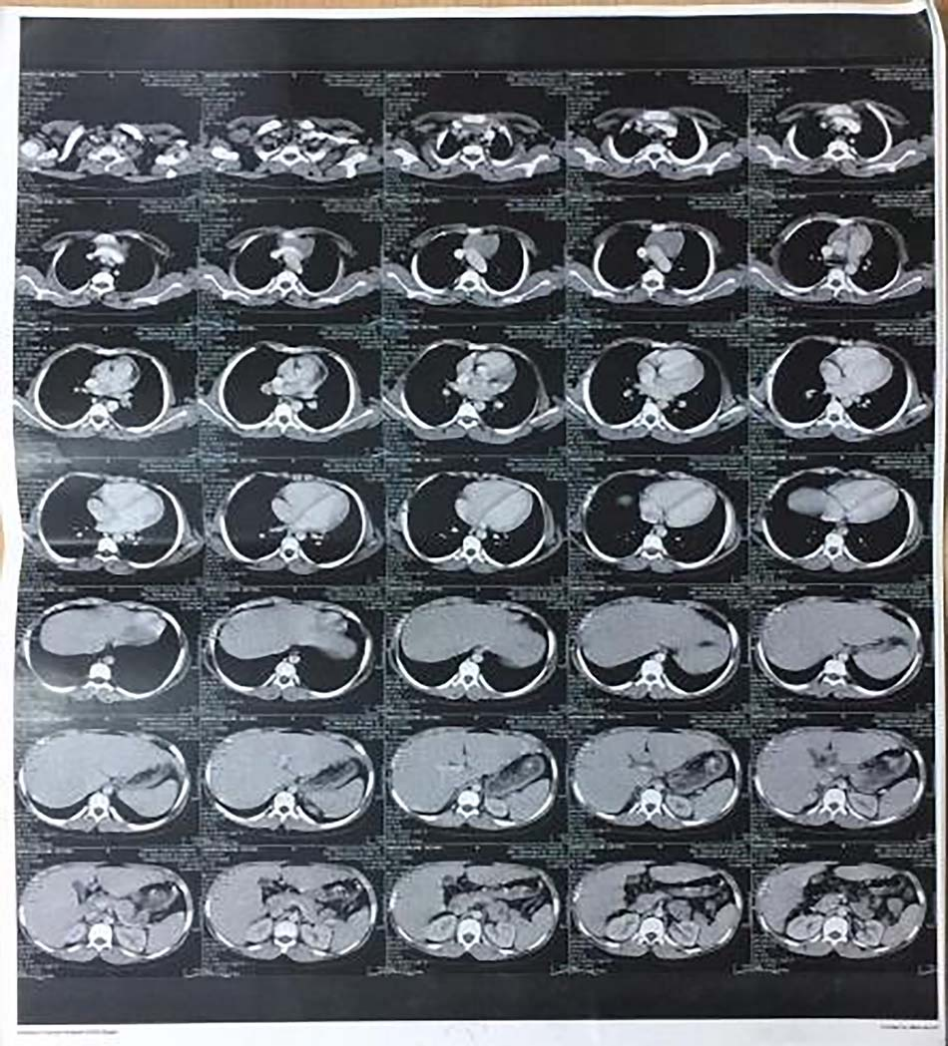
CT of regressed thymic homogenous tissue with clear lung fields; no pleural, pericardial effusion or evidence of enlarged mediastinal or hilar lymph node; the heart and great vessels were normal.
